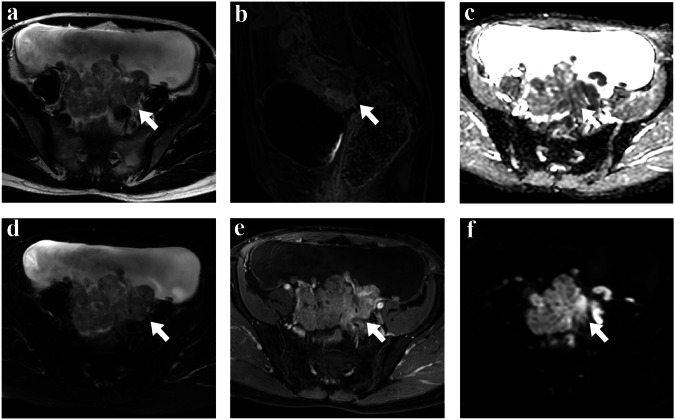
The MR images of a 54-year-old non-CCC (high-grade serous carcinoma) female (FIGO IVB stage) with massive ascites were shown as follows. Axial T2WI and axial FS-T2WI (a, d), demonstrated the left and right ovary had multiple solid nodules fusing into clusters (arrowhead), that had prominent enhancement on CE-T1WI with FS (b, e). Axial DWI showed the solid tissues had high signal (f), while they had low signal at ADC map (c)
